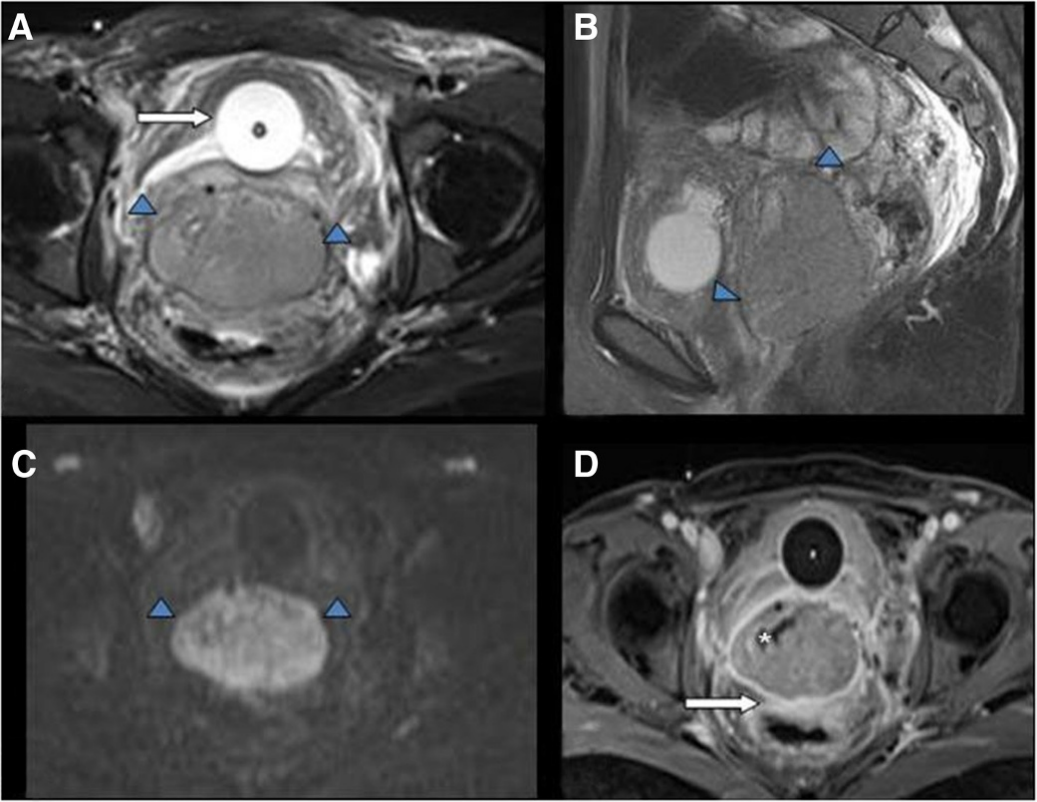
MRI scans for the recurrent tumor. (A) Axial fat-suppressed T2WI; (B) Sagittal fat-suppressed T2WI; (C) Diffusion weighted imaging (DWI); (D) Contrast-enhanced fat-suppressed T1WI. In this case, the giant oval mass (arrowhead) with homogeneous isointensity signal was well demonstrated on T2WI (A, B). Note, the balloon catheter (arrow) was placed into the bladder. On DWI (C), the tumor (arrowhead) appears hyper-intensity signal compared with hypointensity signal of background. On contrast-enhanced MRI (D), the tumor mildly enhanced and the necrosis component (*) did not enhanced. Note, the mass-rectum margin was not clear (arrow), indicating the tumor invaded the anterior wall of rectum.
